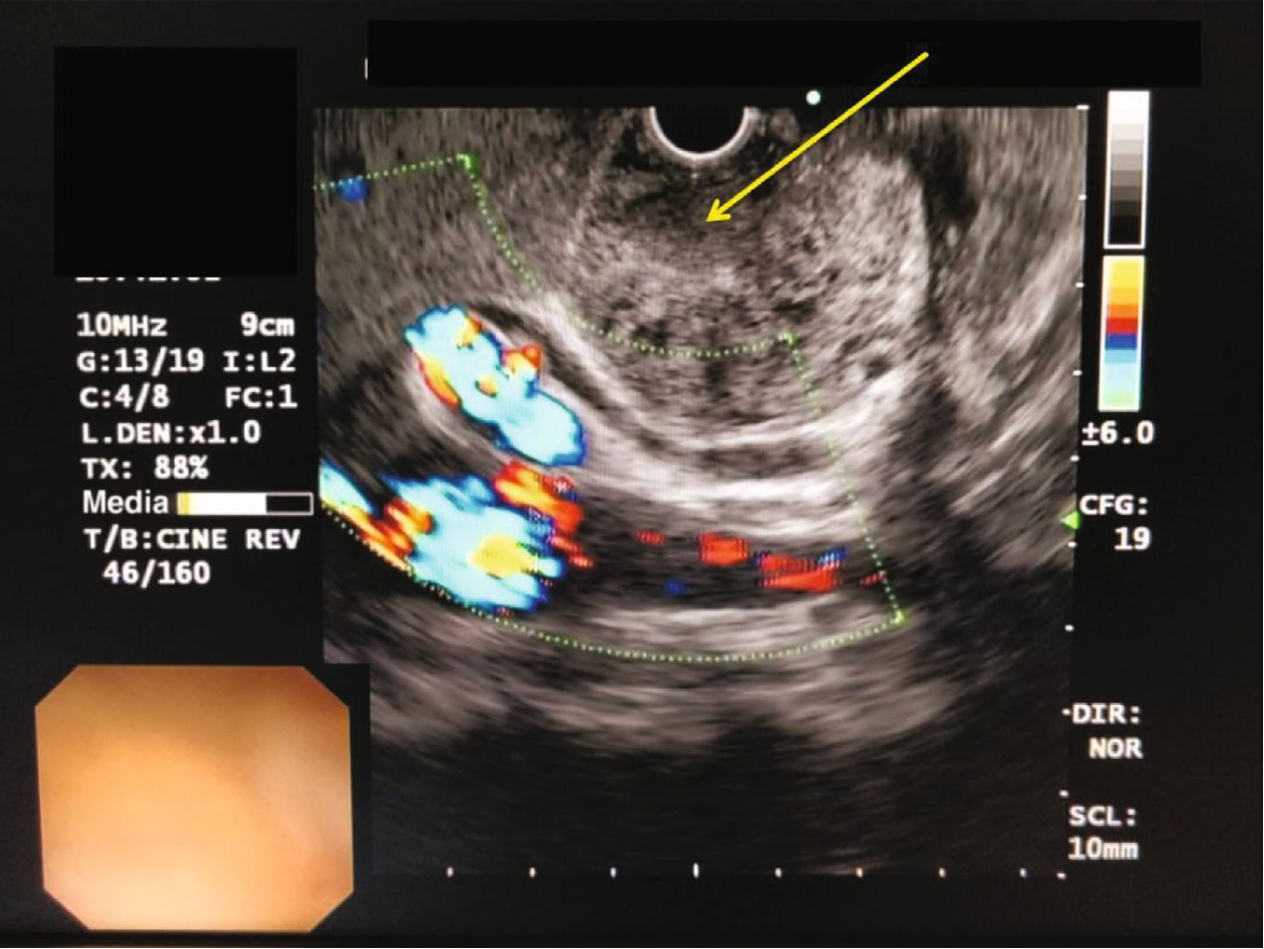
Endoscopic ultrasound (the yellow arrow shows a mixed echogenic collection in relation to pancreatic head and body).
